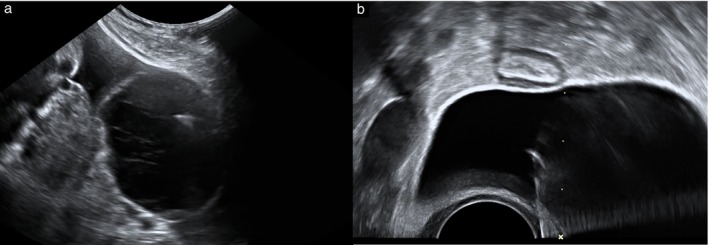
Grayscale ultrasound images showing technique for ultrasound‐guided cyst aspiration via transabdominal (a) and transvaginal (b) route in patients with acute adnexal torsion.
